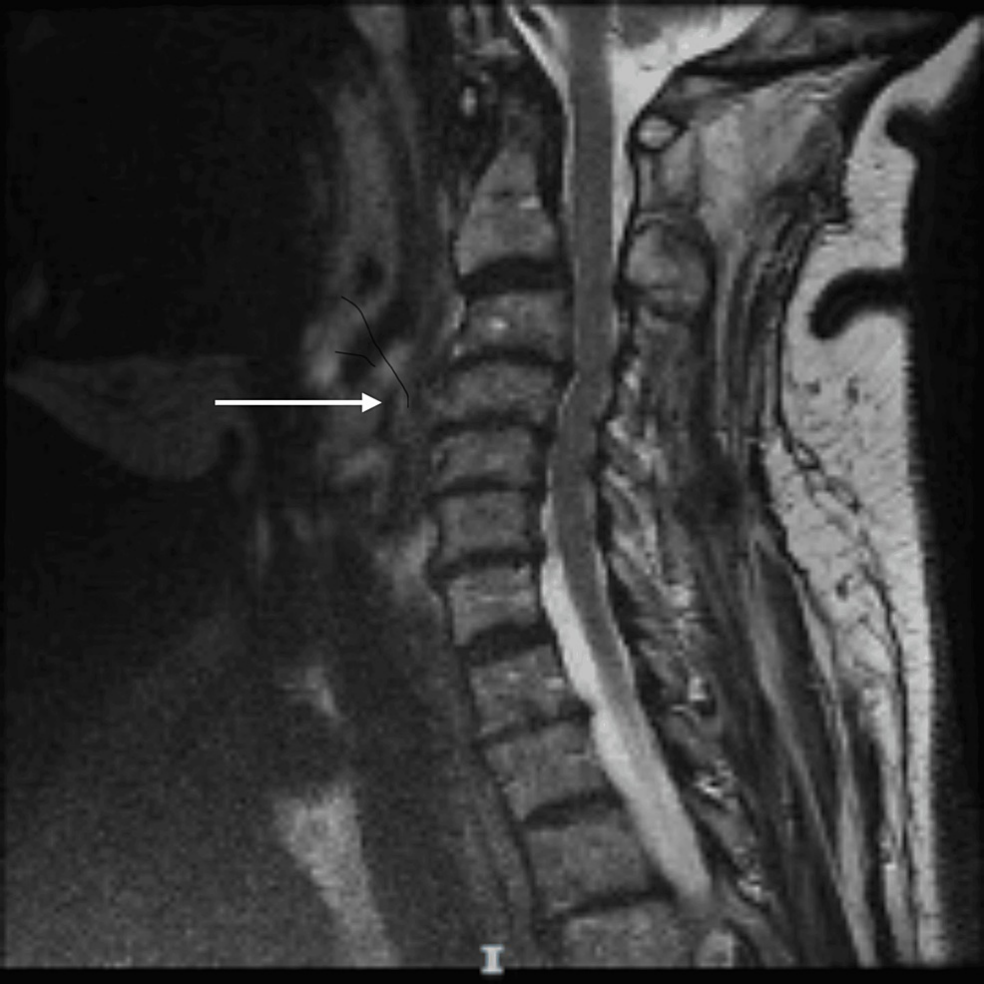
Magnetic resonance imaging of the cervical spine T2-weighted image of the cervical spine depicting multilevel mild disc degeneration with C3/4, C4/5, C5/6, and C6/7-disc osteophyte complexes. These findings are associated with evidence of multilevel spinal canal stenosis.
